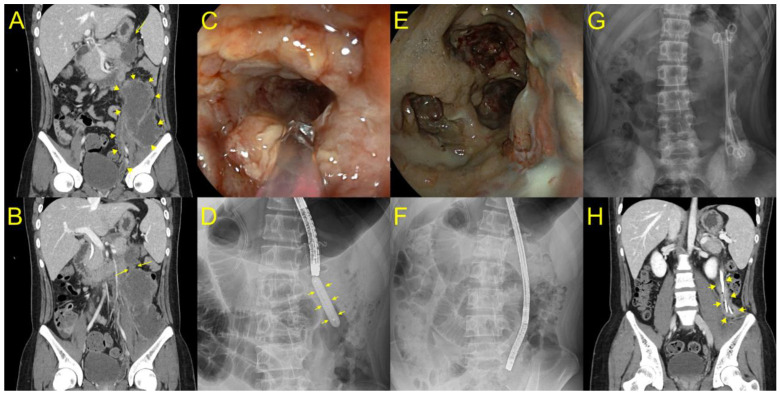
Placement of long plastic stents to the deep portion of walled off necrosis (WON) after direct endoscopic necrosectomy (DEN) for endoscopic treatment of laterally extended WON (yellow arrow) (A). (B) Computed tomography showing a huge WON extended to the left pelvic cavity communicating to a small portion of the necrotic cavity concatenated with the stomach through the narrow neck (yellow arrow) portion. (C) A gastroscope was inserted into the proximal portion of WON through a lumen-apposing metal stent (LAMS), and we found a luminal stricture of the neck portion of WON. (D) Balloon dilatation (yellow arrow) was performed for deep insertion of an endoscope to the laterally extended WON. (E,F) The cavity was lavaged with normal saline, and necrotic debris was progressively removed with snare and grasping forceps. (G) After DEN, three plastic stents with double pigtail configuration were fixed in place through the LAMS to the deep portion of WON until the next session of DEN. (H) After 16 DEN sessions, where the long plastic stents remained in the deep portion of WON, the patient recovered with complete WON resolution (yellow arrow).
